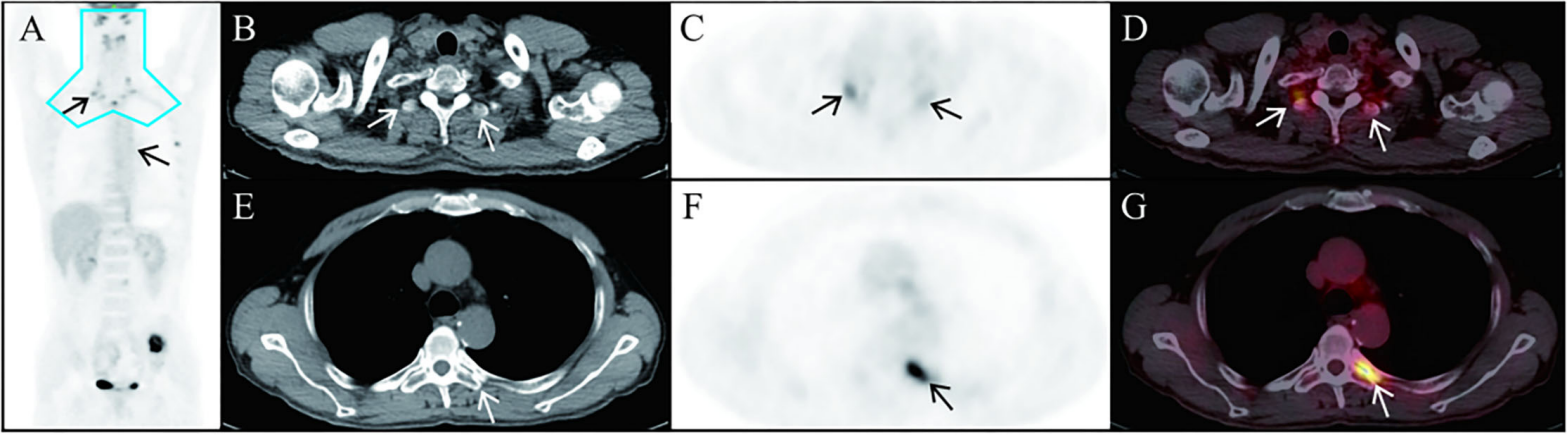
In this case, 18F-FDG uptake (SUVmax, 6.5) is seen in the bilateral vertebrae and intercostal muscles. Representative brown adipose tissue in the thoracic vertebra transverse process [(B–D), arrows] and in posterior intercostal space [(E–G), arrowhead] in the axial CT, PET, and fusion PET/CT images is exhibited. The most common location for brown adipose tissue that is detectable in adults by PET/CT is the blue area in (A).
